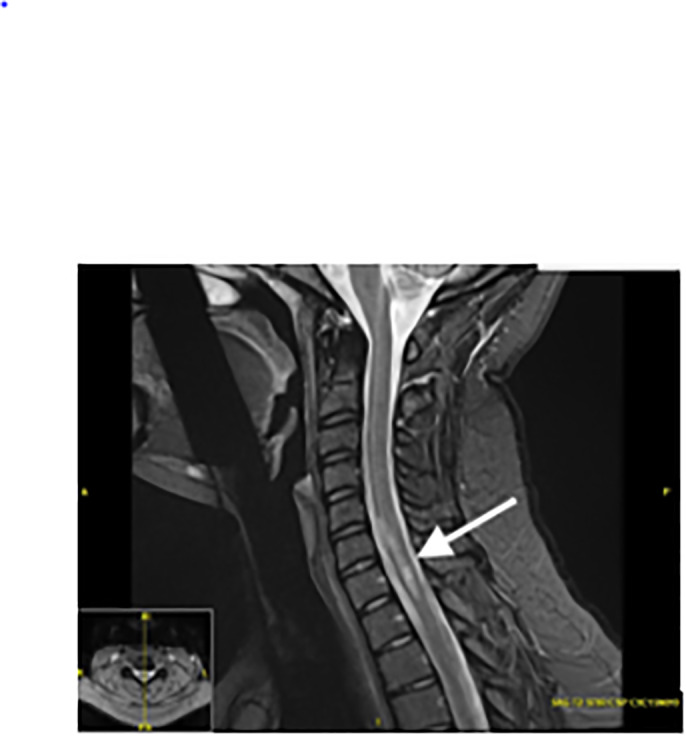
Sagittal T2-weighted magnetic resonance imaging with asymptomatic hyperintensity within the cervical spine, worse at cervical level 5–7 (arrow).
